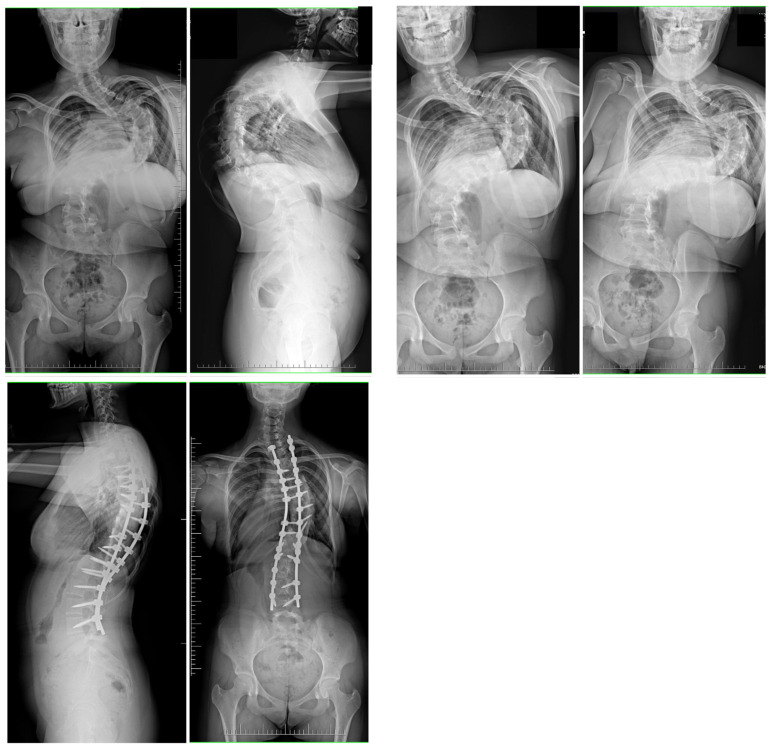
A 14-year-old girl with severe adolescent idiopathic scoliosis treated with preoperative Halo gravity traction followed by multilevel Ponte osteotomies and final correction with double Co-chr 6.0 rods. Pre- and postoperative radiographs during observation period.
